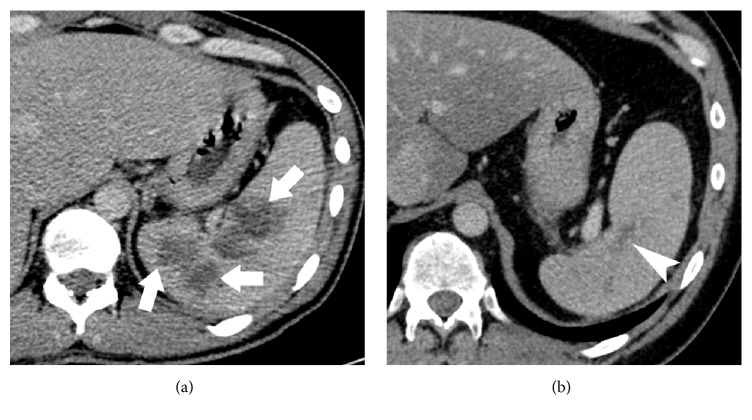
Axial contrast enhanced CT images demonstrating (a) multiple splenic abscess (thick arrows) with (b) resolution and residual scarring (thin arrow) following treatment with steroids.
